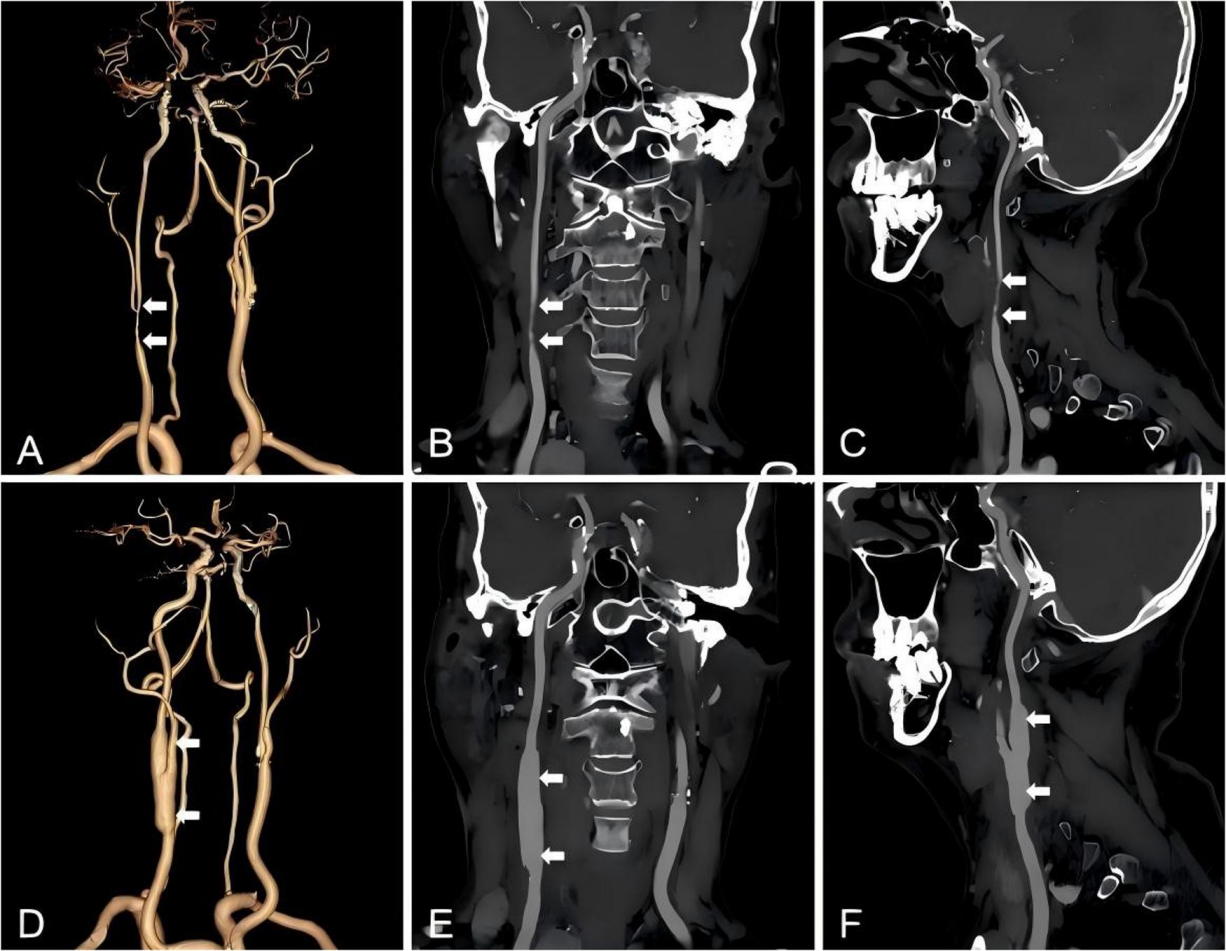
CTA images before and after the second CEA. Three-dimensional CTA images prior to reCEA, and white arrows point to the location of restenosis. (A) Coronal and sagittal CTA views show restenosis development in the right internal carotid artery following the initial CEA (white arrows). (B–C). Three-dimensional CTA images following CEA combined with patch angioplasty, with white arrows pointing to the surgical site and the diameter of the blood vessels was increased. (D) Coronal and sagittal CTA sections show improved luminal patency and resolution of restenosis after operation (white arrows). (E–F).
